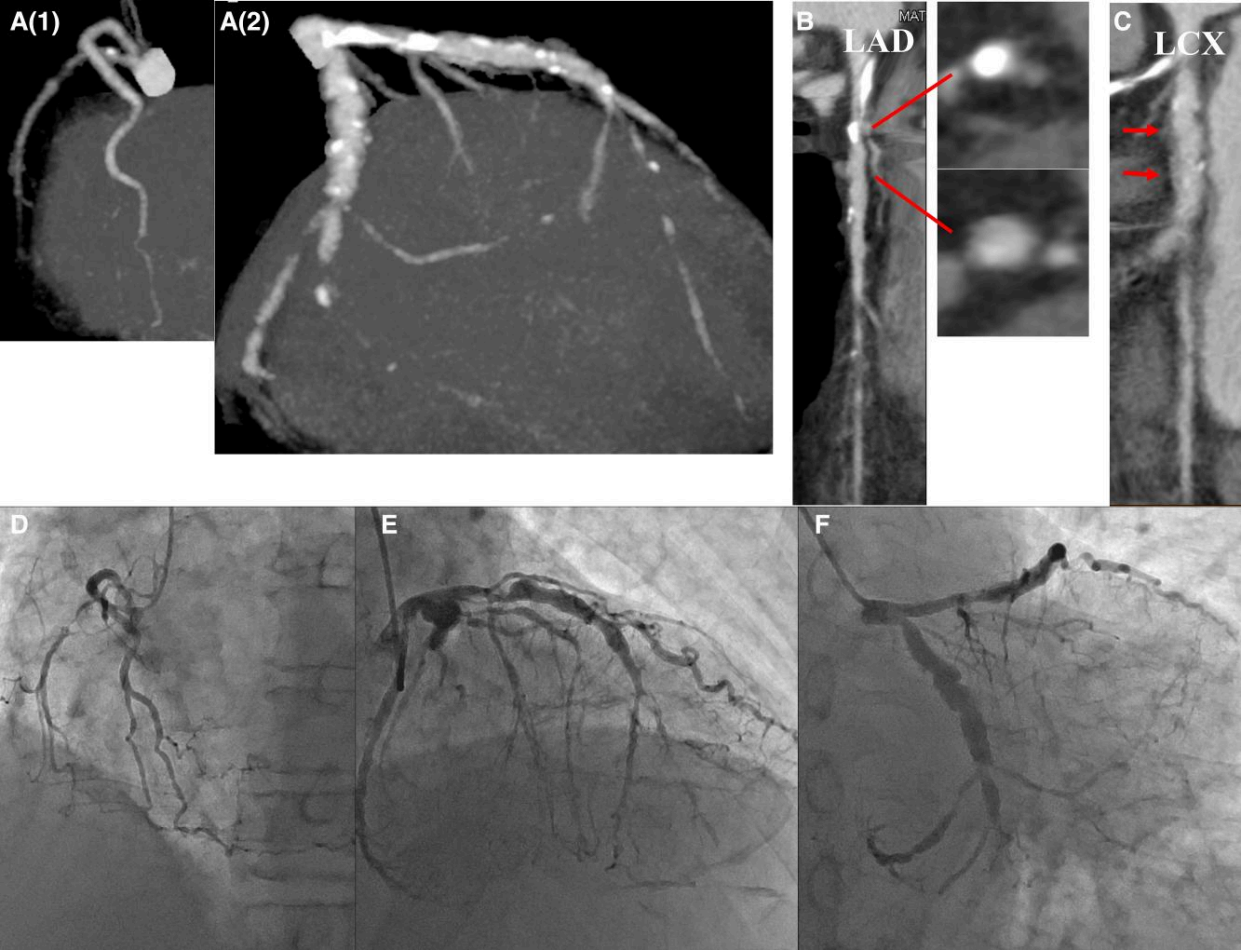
Coronary artery imaging. Coronary computed tomography angiography shows hypoplasia in the right coronary artery (*A*1) and a mixture of dilated lesions and stenosis in the left coronary artery (*A*2). (*B*) The short axes of the calcified lesion and coronary aneurysm in the left anterior descending artery are shown (arrows). (*C*) In the left circumflex artery, diffuse perivascular soft tissue thickening is observed (arrows). Coronary angiography shows a severe three-vessel disease with aneurysmally dilated lesions and stenosis (*D*, *E*, *F*).
